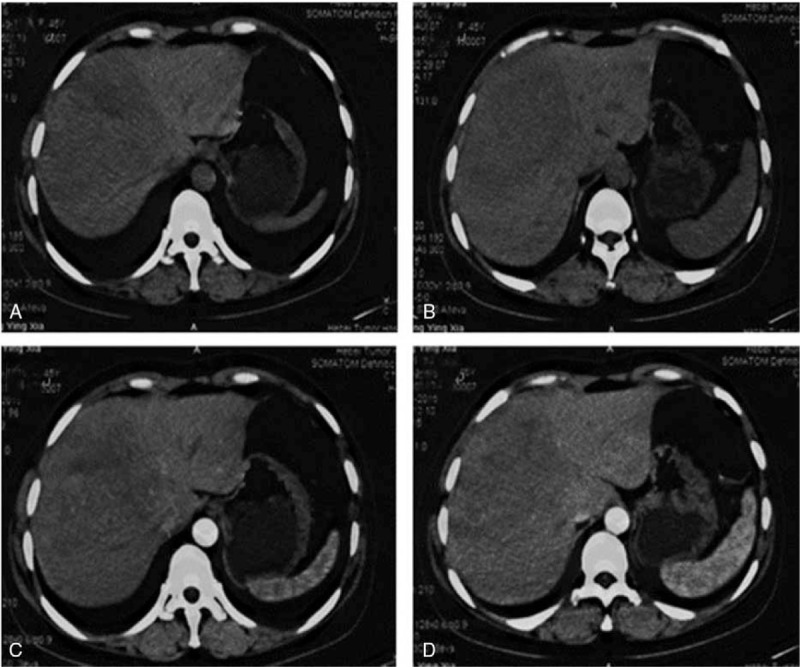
CT: (A, B) Plain phase. A tumor approximately 11.0 cm in diameter was found in the right lobe of the liver; (C, D) Arterial phase. The peri-tumor area was slightly enhanced.
